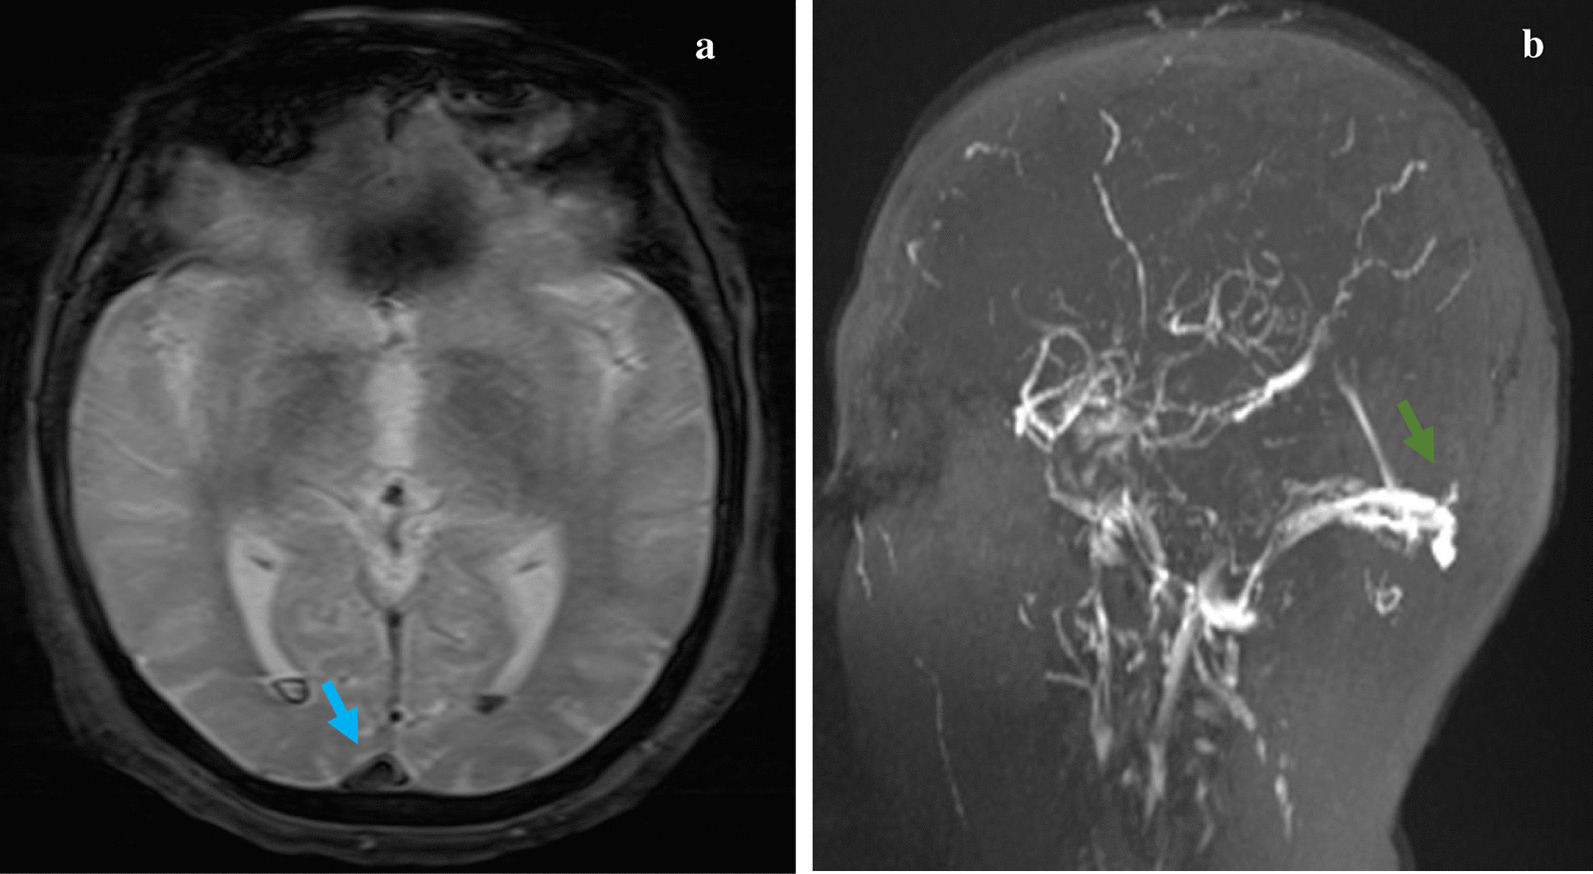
a Axial T2*GE magnetic resonance imaging showing a low-attenuating thrombus within the superior sagittal sinus with “empty-delta” sign (blue arrow) and hypointense areas in occipital horns of both lateral ventricles corresponding to subarachnoid hemorrhage. b Sagittal magnetic resonance venography showing the filling defect in the superior sagittal sinus indicative of Superior Sagittal Sinus Thrombosis (SSST) (green arrow)
